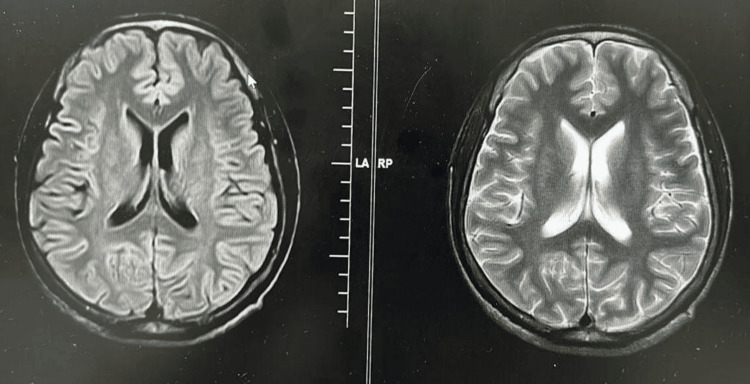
MRI of the brain showing no abnormalities
